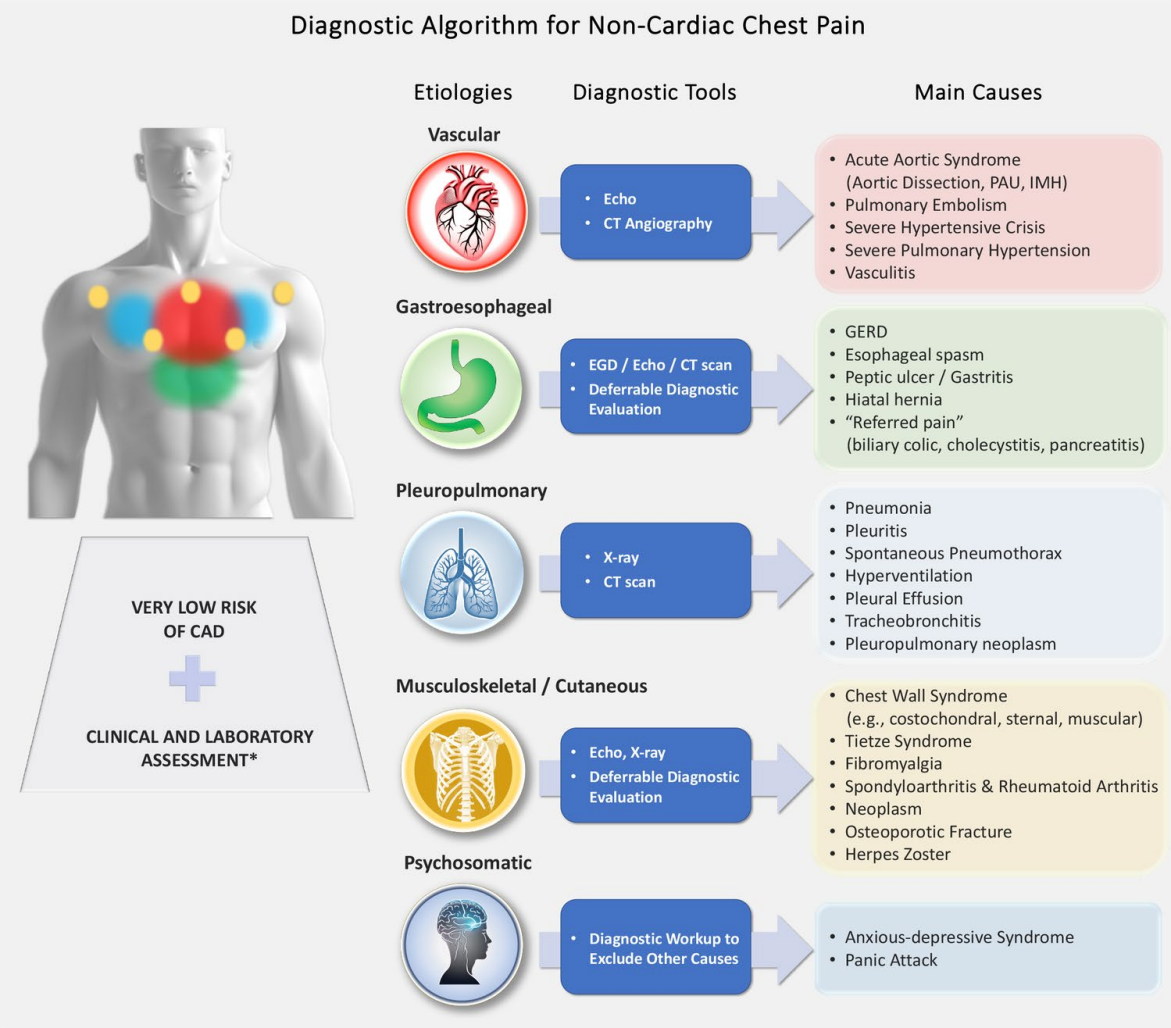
Diagnostic Algorithm for Non-Cardiac Chest Pain. *Abbreviations* CAD, coronary artery disease; CT, computed tomography; EGD, esophagogastroduodenoscopy; GERD, gastroesophageal reflux disease; IMH, intramural hematoma; PAU, penetrating atherosclerotic ulcer; X-Ray, radiography; Echo, Ultrasound as appropriate (e.g., cardiac, abdominal, vascular, musculoskeletal). *: includes the measurement of cardiac biomarkers and D-dimer, complete blood count, and inflammatory markers
